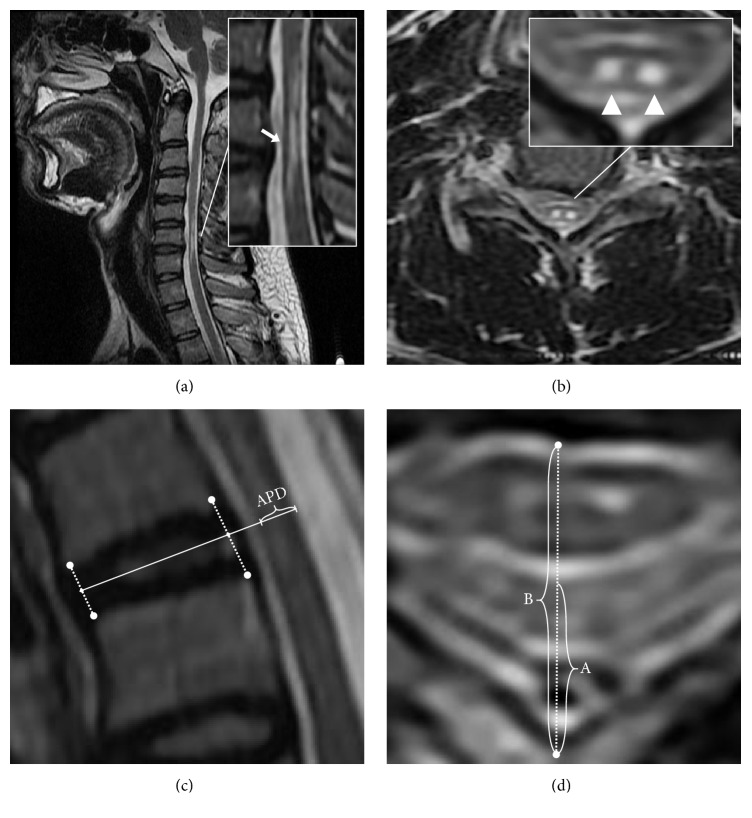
Typical SEA and measurement method on MRI. (a) On the sagittal T2-weighted MRI, the SEA showed a high signal in the middle of the spinal cord. (b) On the axial image, a typical SEA was characterized as a symmetrical, bilateral, small high-signal-intensity lesion. (c) Measurement of anteroposterior diameter (APD) on the sagittal image: APD is the distance between the anterior and the posterior edge of the spinal cord on the mid-disc line. (d) Measurement of spinal cord anterior shifting on the axial image: the distance between the posterior wall of the spinal canal and the posterior/anterior edge of the cervical spinal cord was termed A/B. Then, the ratio of A to B was used as an indicator of anterior shifting.
